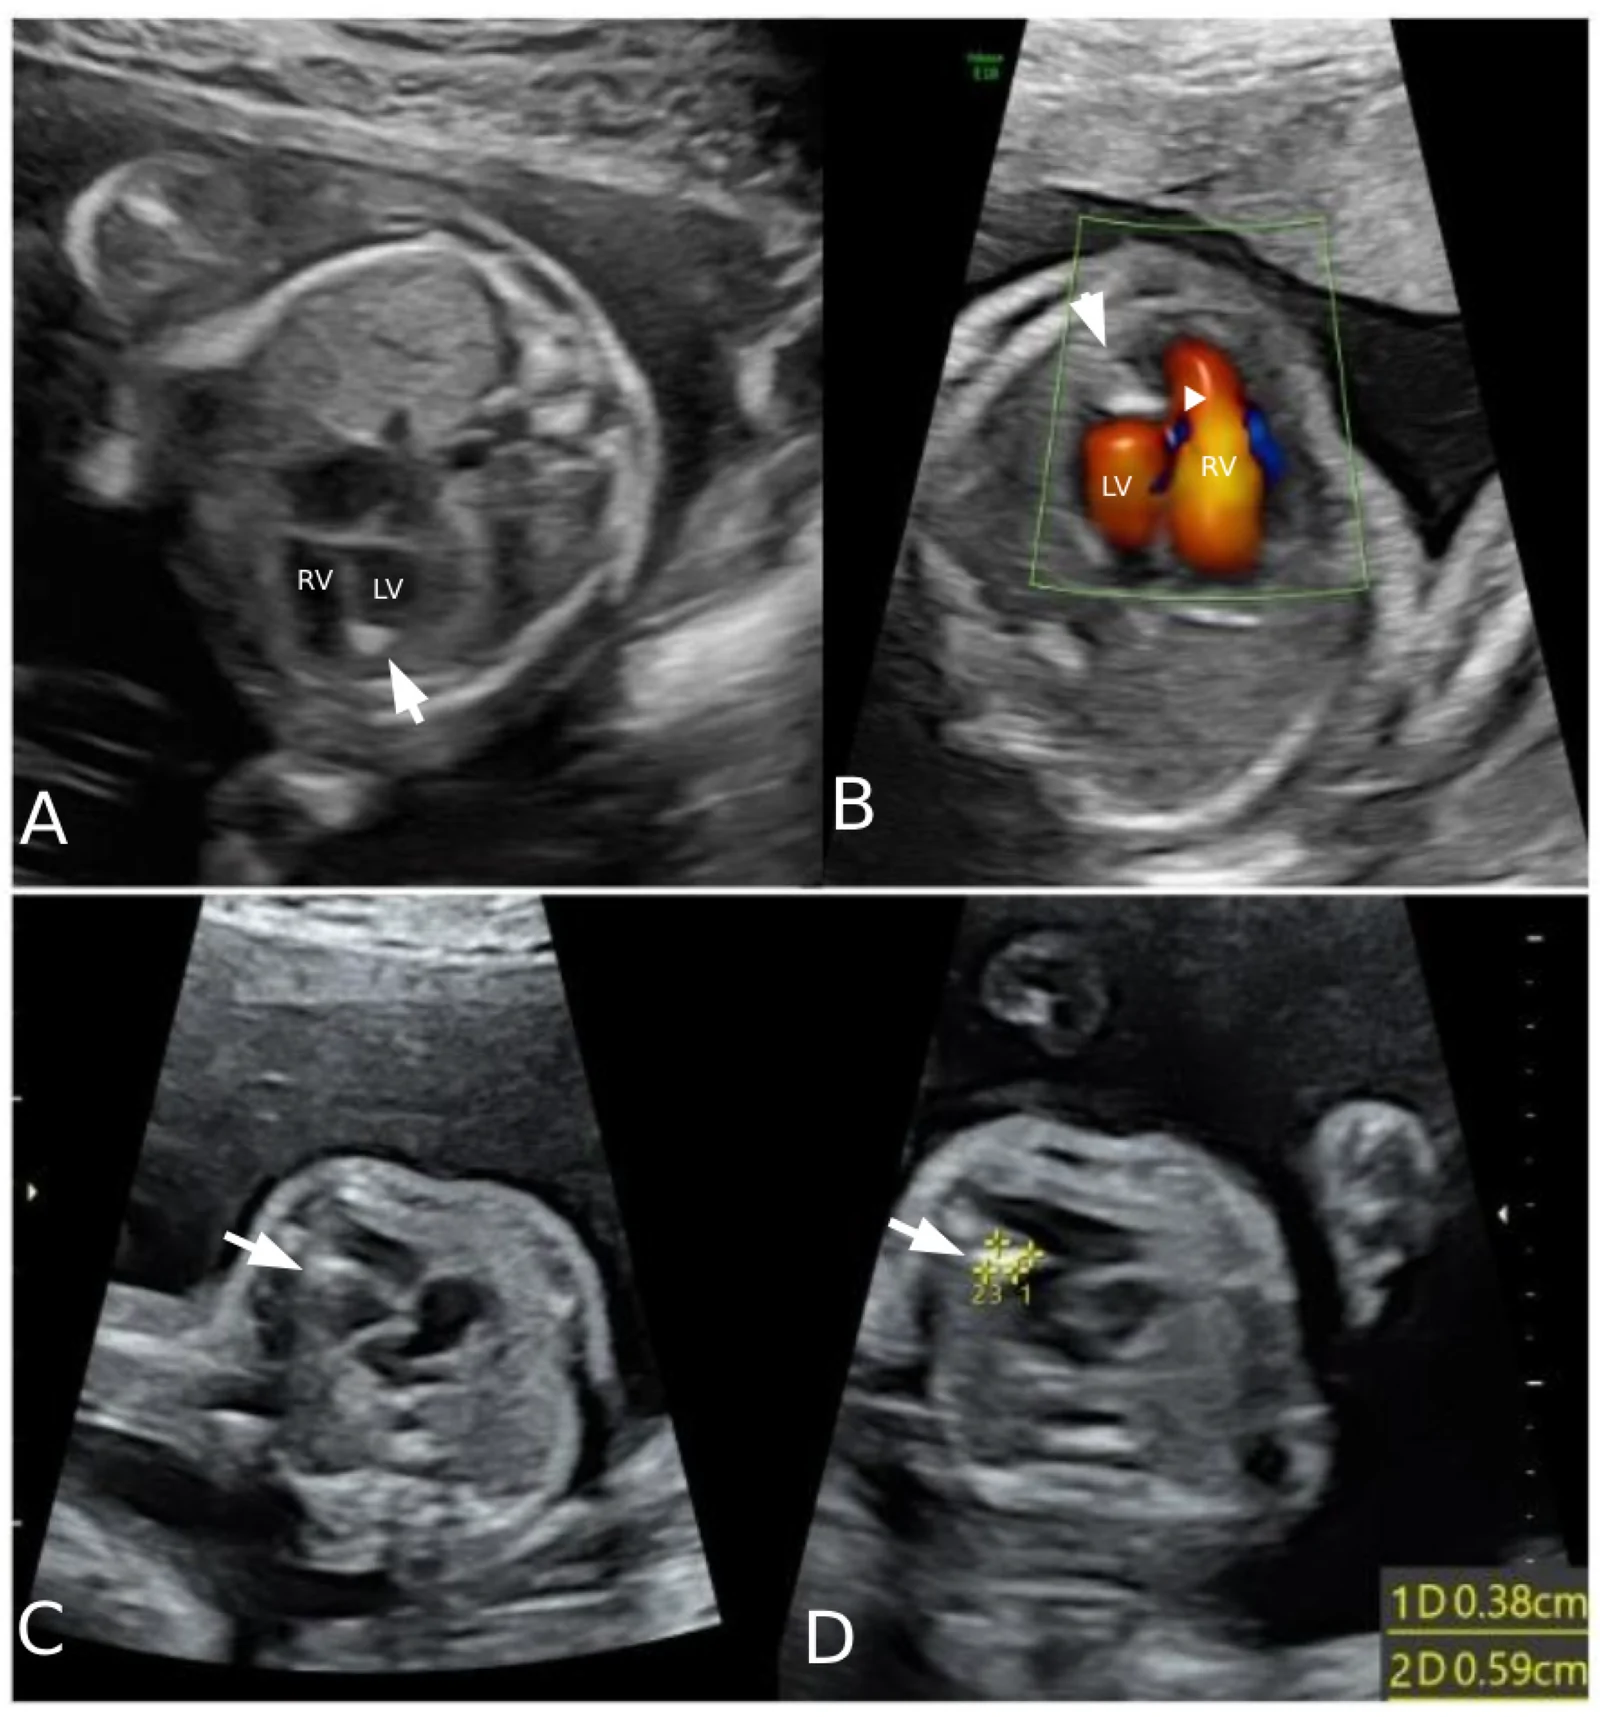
(A) Four-chamber view at 20 weeks of gestation revealing a distinct, highly echogenic triangular mass (white arrow) occupying the anatomical apex of the left ventricle (LV). (B) Color Doppler evaluation at 20 weeks: the LV intracavitary flow abruptly ceases proximal to the space-occupying lesion (white arrow), leaving the apex unfilled. Notably, the right ventricular (RV) apex exhibits normal flow and lacks the characteristic “banana-shaped” elongation at this early stage. A small apical ventricular septal defect (white triangle) can be seen. (C) Four-chamber view at 27 weeks of gestation showing the persistence of the apical lesion (white arrow) and the initial signs of ventricular remodeling. (D) Detailed measurement (yellow crosses) of the focal apical mass at 27 weeks (approximately 3.8 × 5.9 mm); the lesion appears increasingly integrated into the adjacent myocardium.
